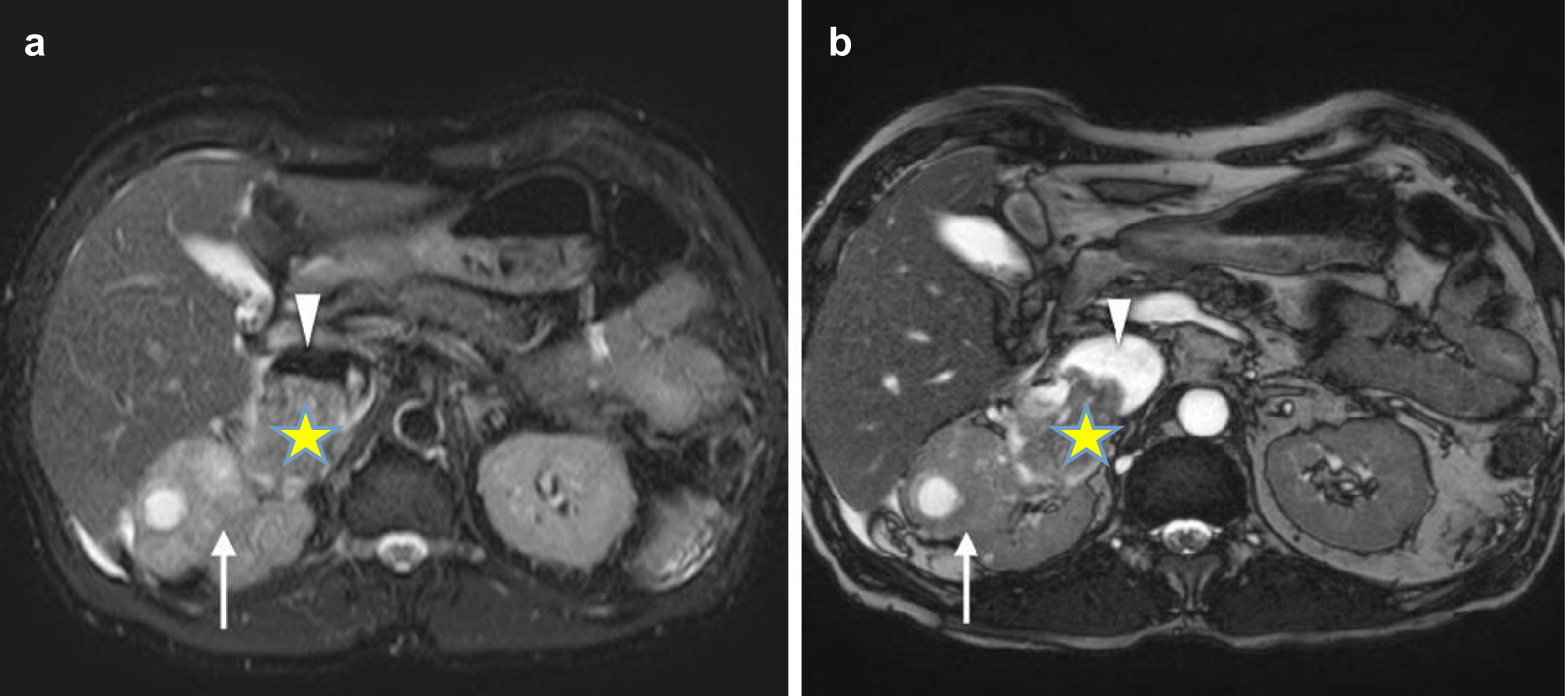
Axial T2-weighted fat saturation (a) and balanced turbo field echo (b) images showing the heterogenous right renal mass with cystic component (*arrow*) and formation of tumor thrombus in the dilated right renal vein (*yellow asterisk*) which protrudes into the intrahepatic inferior vena cava (*white triangle*)
